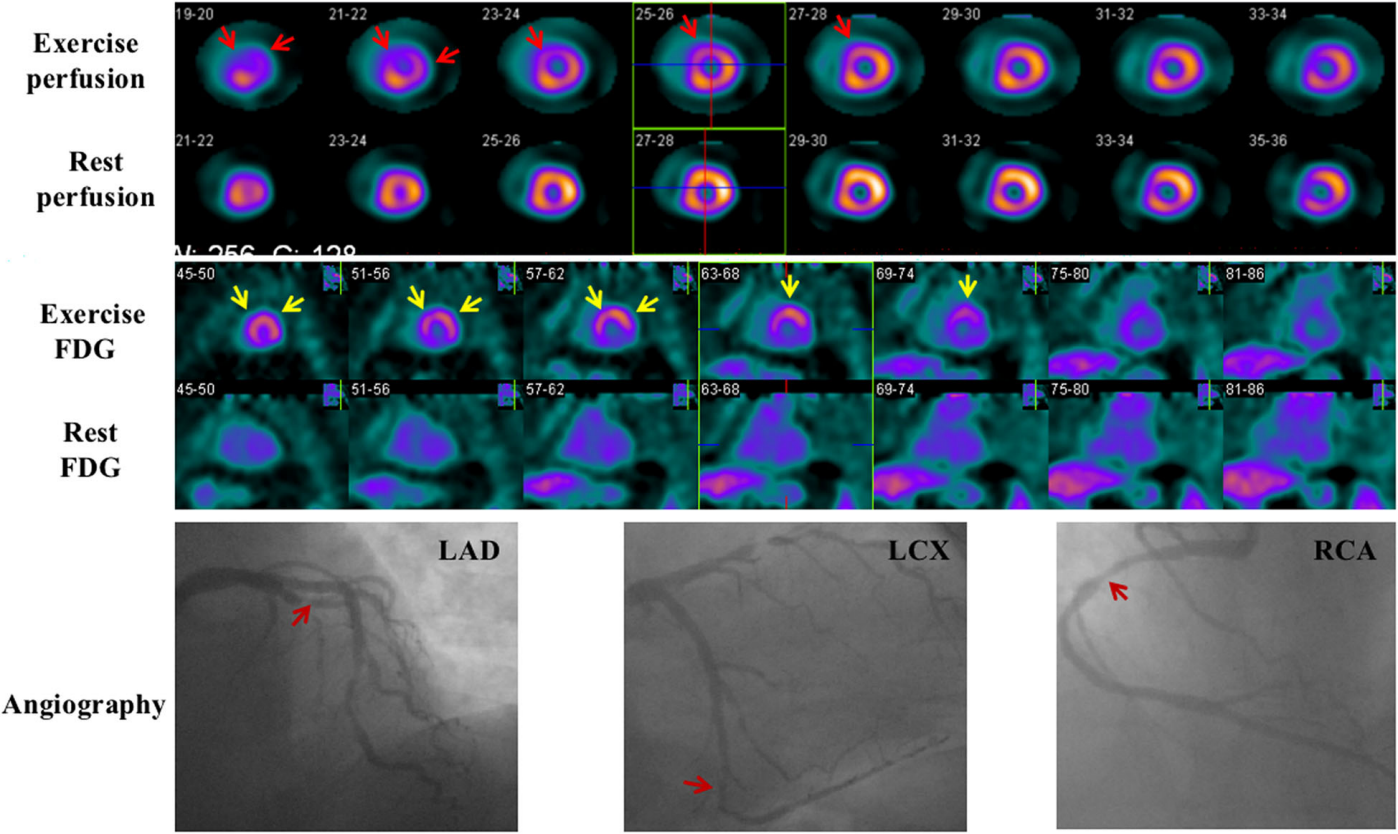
Images of a 61-year-old woman. She had a 99% stenosis in the left anterior descending coronary (LAD), a 90% stenosis in the left circumflex coronary (LCX), and a 70% stenosis in the right coronary artery (RCA). Perfusion images show reversible defects in the anterior and lateral wall (*red arrows*). Resting ^18^F-FDG images indicate only background uptake in the cardiac cavities and no visible uptake in the left ventricular wall (‘none’ pattern). Nevertheless, exercise ^18^F-FDG images exhibit intense uptake in the anterior and lateral wall (‘focal’ pattern, *yellow arrows*) which is in accordance with exercise perfusion images
